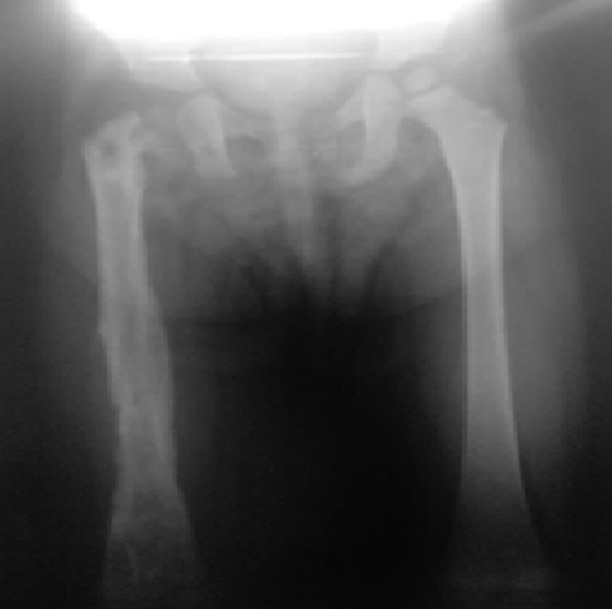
radiographie standard du fémur droit prenant le fémur controlatéral: ostéomyélite aiguë du col du fémur droit compliquée d´une pandiaphysite chronique avec raccourcissement fémoral et varisation du col
